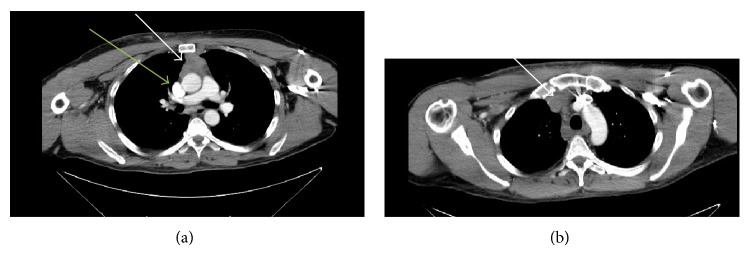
CT chest with contrast: 6/29/2014. Technique: contiguous transaxial images were obtained from the thoracic inlet to the upper abdomen with contrast. 125 cc omnipaque 350. (a) Thrombosis of the right internal jugular vein with extension to the right innominate vein and subclavian vein was noted (green arrow). The origin of the great vessels was patent. There was an anterior mediastinal mass (white arrow) present measuring 4.4 × 3.4 cm. Fibrotic changes were noted at the right base. There was no pulmonary mass or infiltrate. The thin cut after contrast series demonstrated no evidence of large pulmonary emboli. Fluid attenuation was noted just superior to the azygos arch likely representing prominence of the pericardial recess. There was a 2.8 × 1.1 cm lymph node in the subcarinal region image. There were prominent right axillary lymph nodes noted. (b) There was prominent soft tissue attenuation in the anterior mediastinum which was abutting the thrombosed jugular and innominate veins (white arrow). This was likely superior extension of the previously mentioned anterior mediastinal mass.
